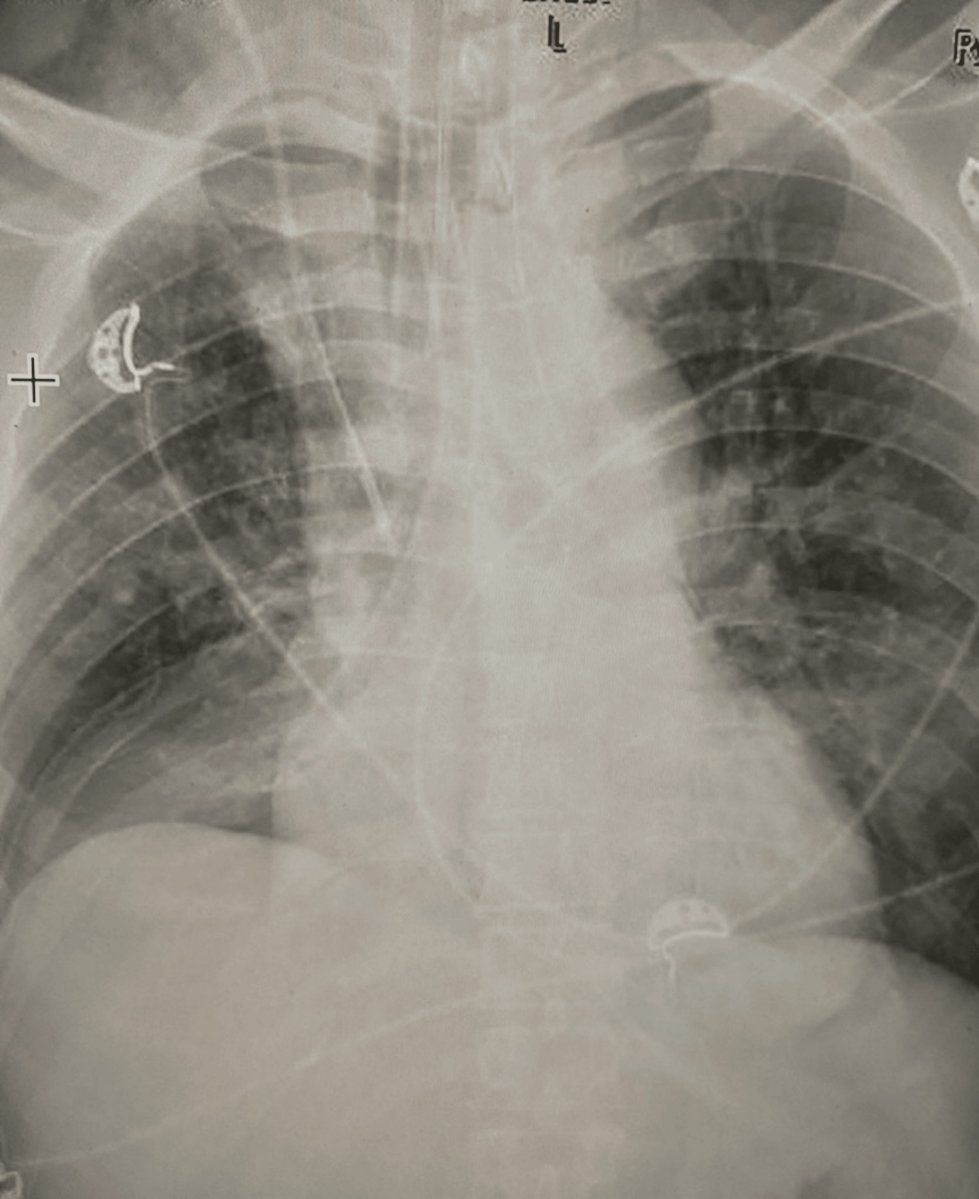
Chest X-ray showing nasopharyngeal airway in the right bronchus.
